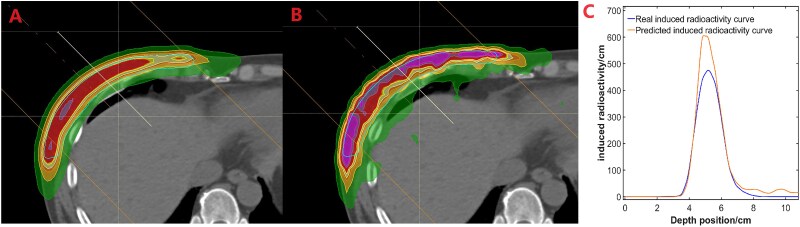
The comparison between the predicted and measured PET images. The profile was obtained from the localization CT image. The irradiation was conducted from the upper left corner towards the lower right direction, with the thick solid line in the image representing the field angles. Panel A shows the predicted distribution of positron emission, while panel B presents the measured distribution. Panel C exhibits the positron emission curves obtained by sampling along the thick white line from the predicted and measured images, which are used for calculating ΔR50. As can be observed in the figure, there are differences between the predicted and measured images in some regions. The emission values at the same spatial locations can be compared using the sampling lines, and the errors can be calculated accordingly.
